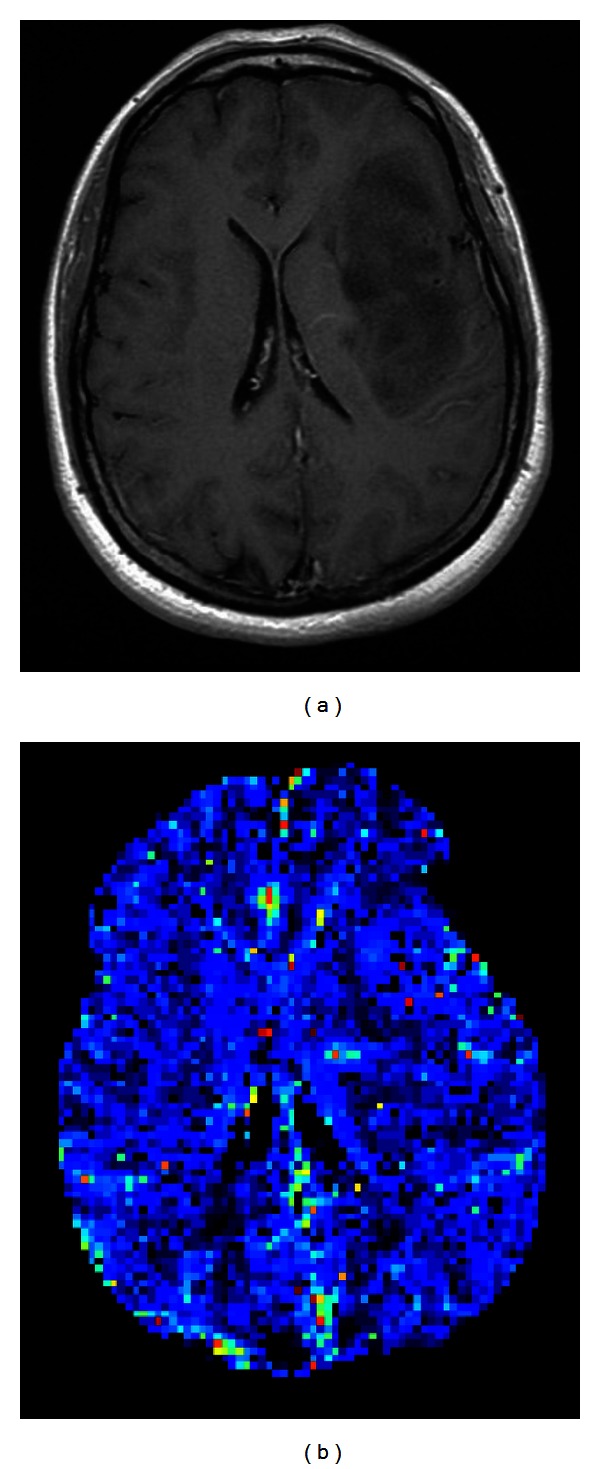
(a) Contrast-enhanced T1-weighted image depicts a nonenhanced astrocytoma, WHO grade II. (b) The *K*
